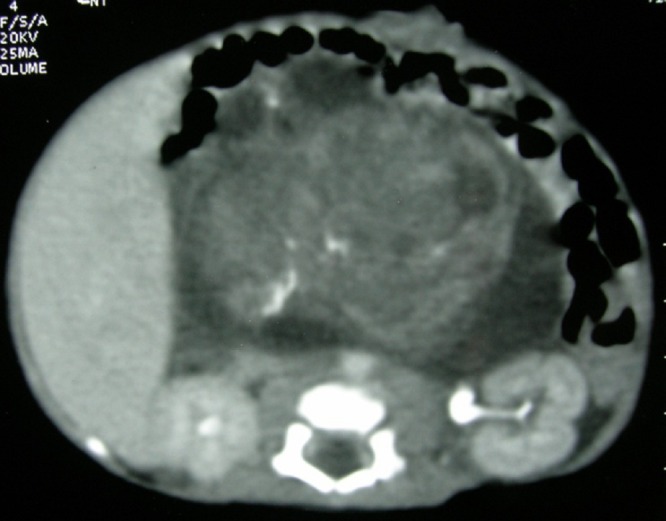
Figure 1: CT scan abdomen showing a large heterogeneous retroperitoneal mass with foci of calcification.
